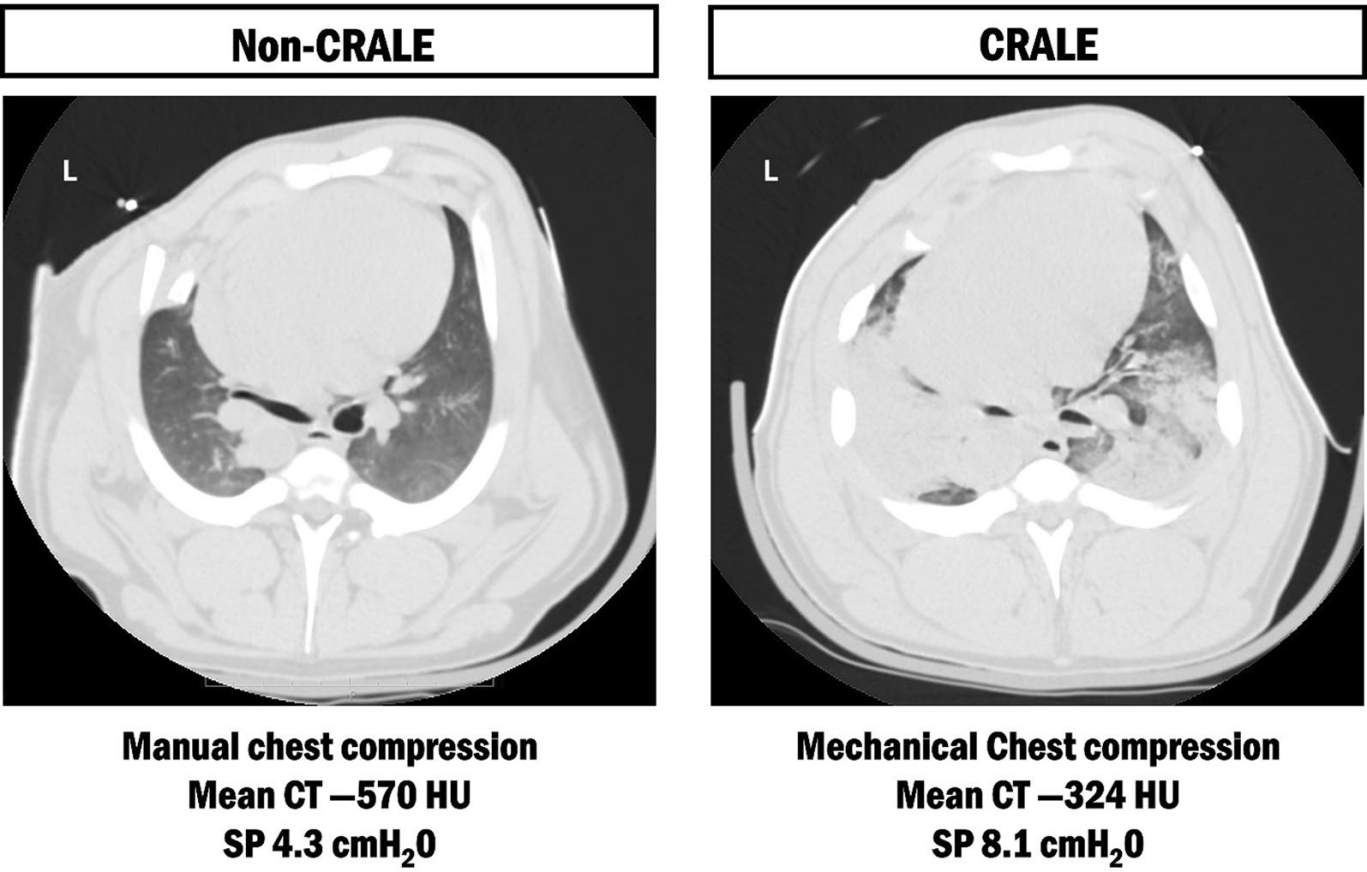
Representative axial chest CT images after cardiopulmonary resuscitation with different compression modalities. Left: manual chest compressions, without evidence of cardiopulmonary resuscitation-associated lung edema (CRALE), with lower mean lung density and preserved aeration. Right: mechanical chest compressions, with CRALE, characterized by higher mean lung density (higher mean HU values), increased non-aerated lung tissue, and higher superimposed pressure (SP)
